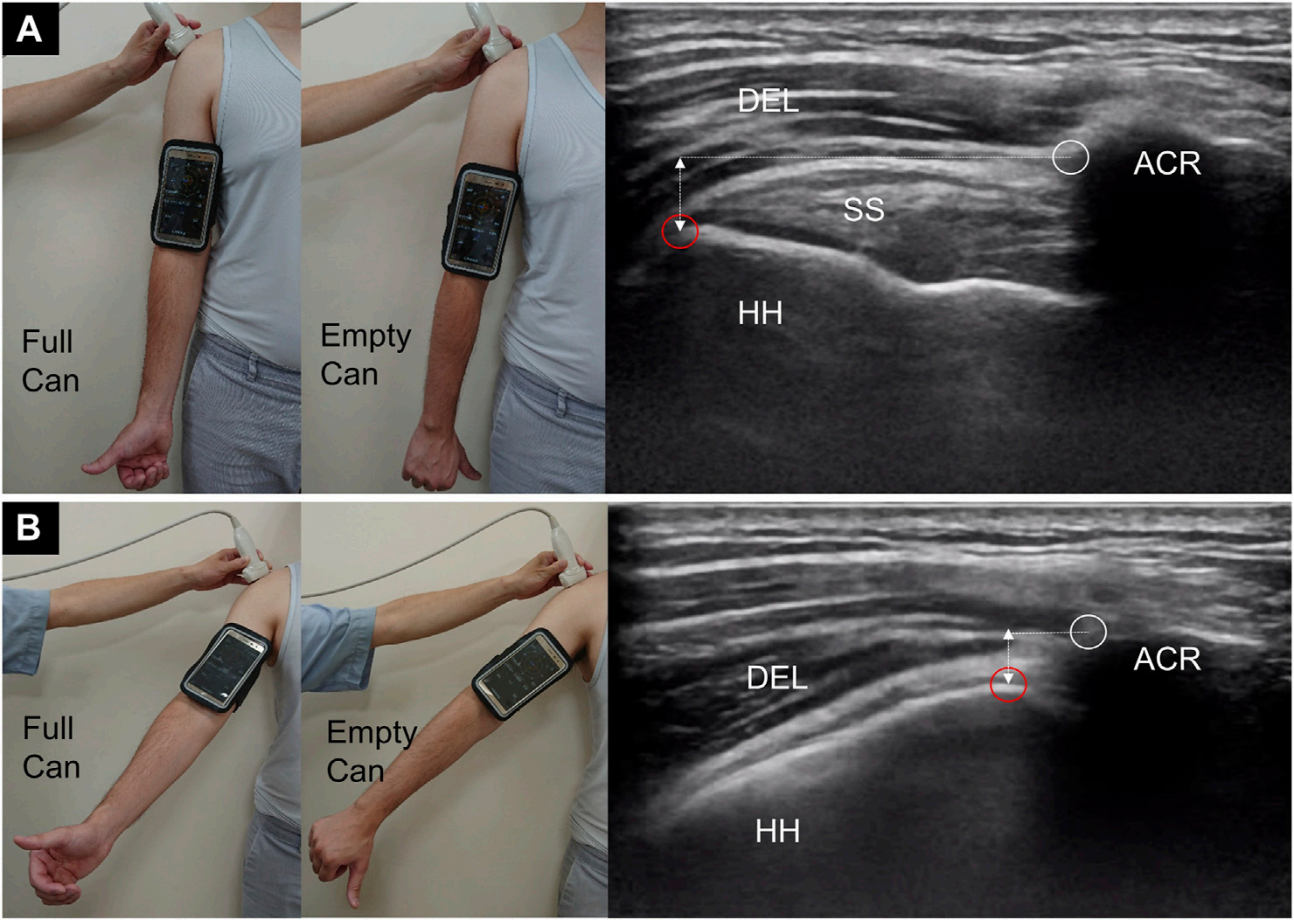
The posture of the upper extremity and ultrasound imaging of the subacromial region at the starting (A) and abducted (B) position. In (B), the greater tuberosity (red circles) is about to pass the lateral acromial edge (white circles). DEL, deltoid muscle; HH, humeral head; SS, supraspinatus tendon; ACR, acromion; double-head dashed line, vertical acromiohumeral distance.
